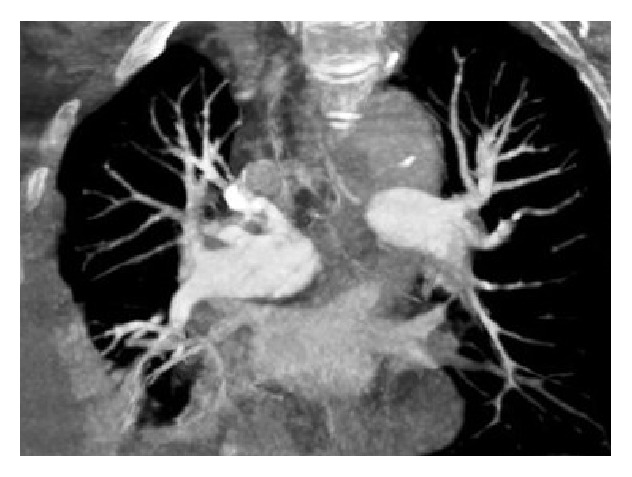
Computed tomogram shows right pleural effusion, web-like filling defects, and endoluminal thromboemboli extending into the right and left pulmonary artery.
